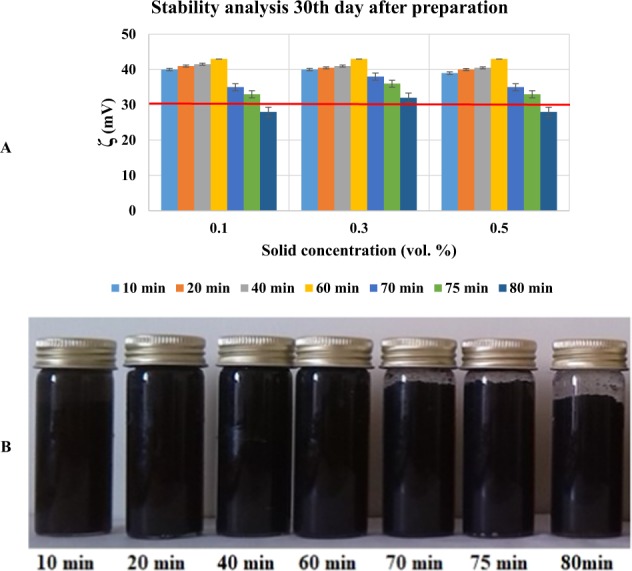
Results of the stability analysis. (A) Zeta potential analysis versus solid concentration for different ultrasonication times, and (B) visual observation versus ultrasonication time for a solid concentration of 0.5 *vol*. %^54^. (Reprinted with the permission of Elsevier).
